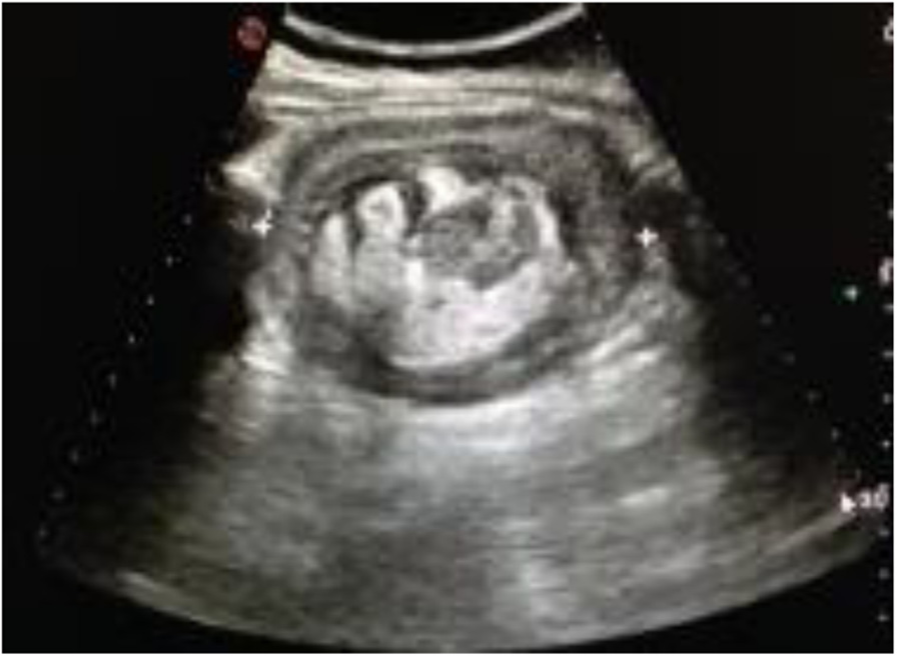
Abdominal ultrasound noted an intussusception, giving the typical “target” sign
